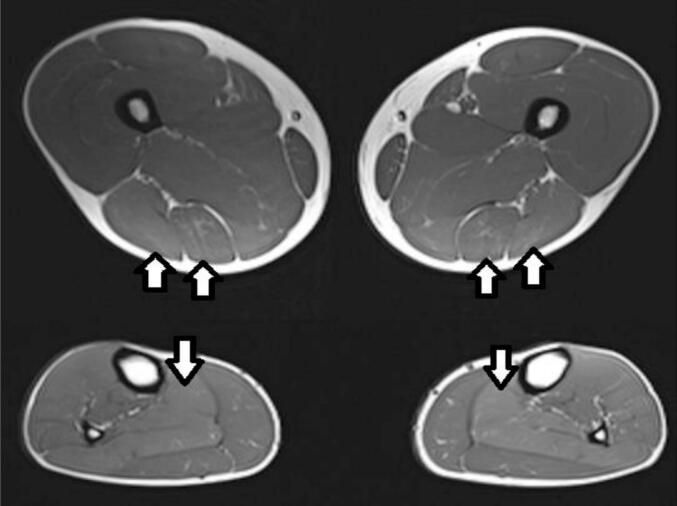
Axial T1 weighted MR images of the thighs (top) and calves (bottom). Subtle homogenous increased T1 signal within the biceps femoris, semimembranosus and soleus muscles bilaterally (arrows).
